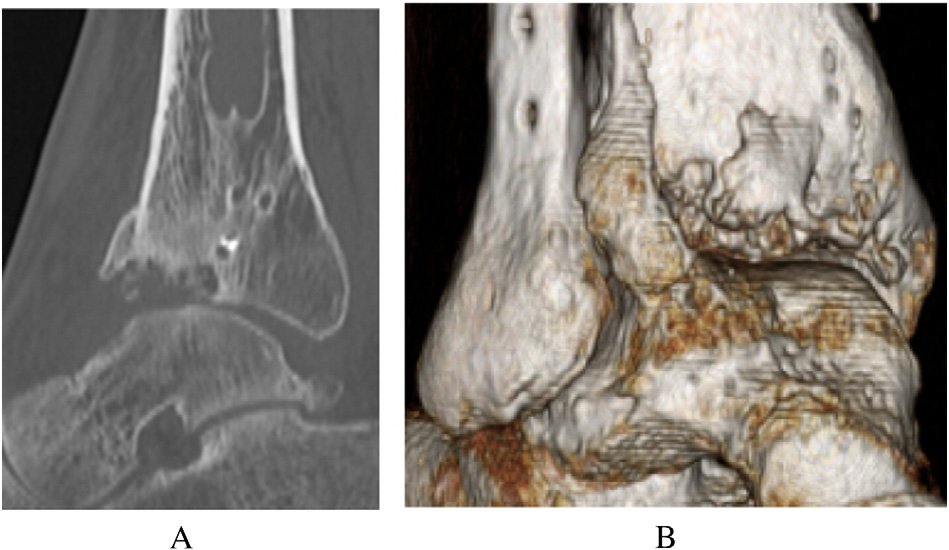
Computed tomography of the right ankle showed the osteochondral defect on the anterolateral surface of the distal tibial plafond, with a surrounding osteophyte: (A) 2D-CT sagittal view, (B) 3D-CT.
